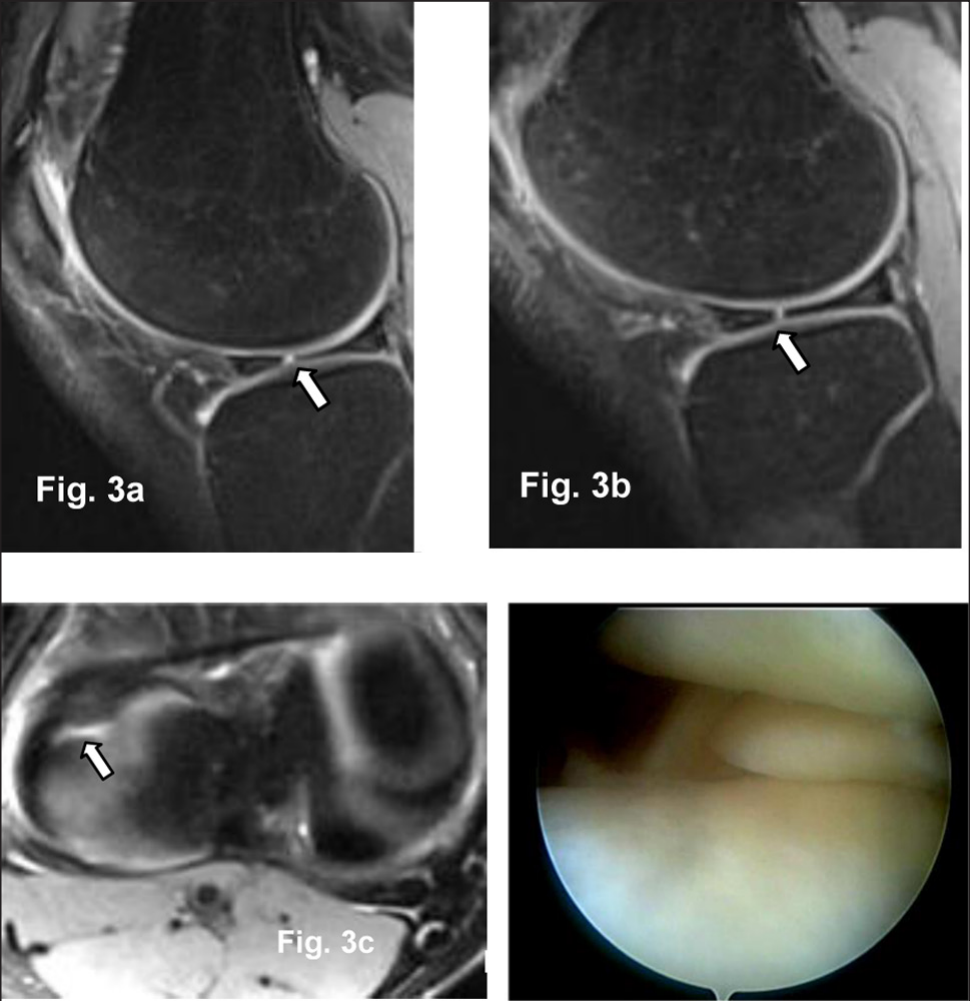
Sagittal (a, b) and transversal 2 mm PD Fat Sat 3T MR images (c): Radial tear in the body of lateral meniscus (arrow) confirmed at arthroscopy (d).
